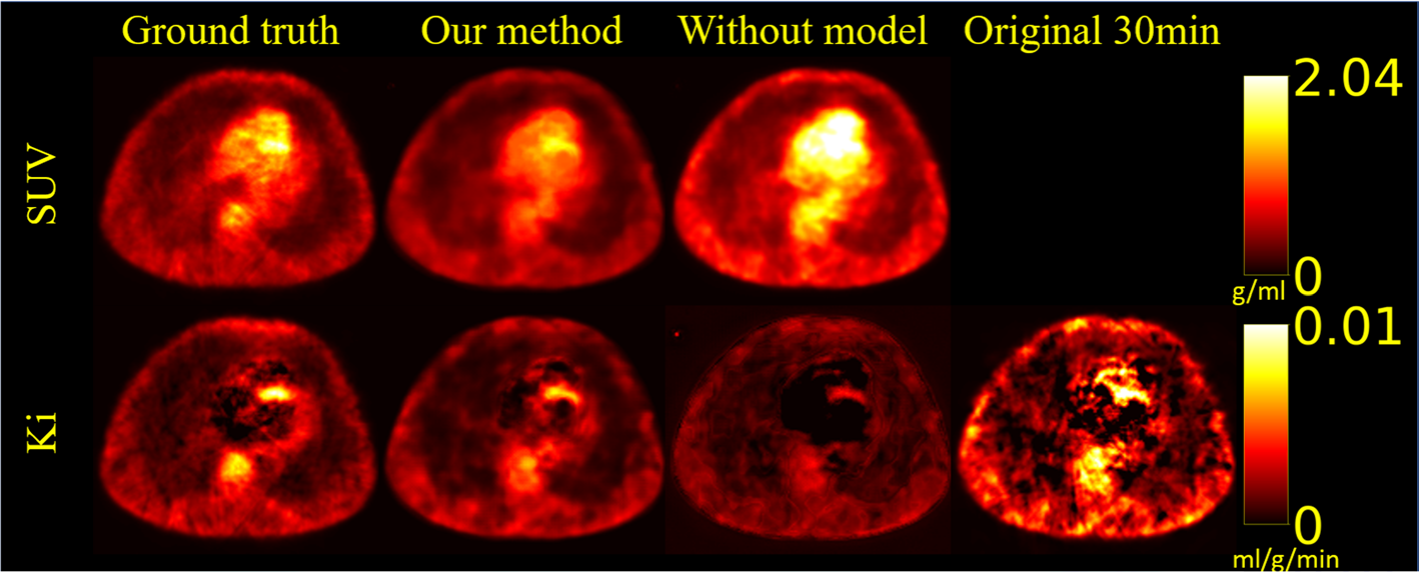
An example of a slice of an SUV image and a slice of a *K*_*i*_ image rendered in pseudocolor
